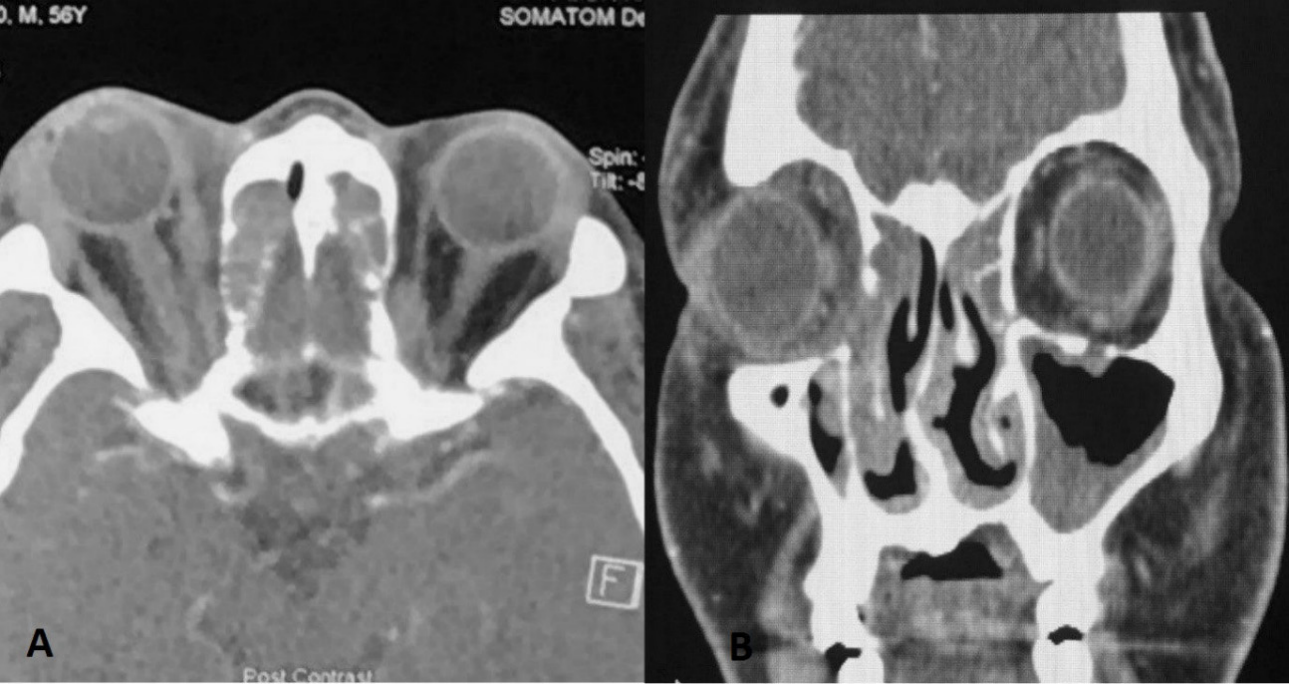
CT scan imaging of the orbit and paranasal sinuses: A) Axial view (left) demonstrated right periorbital soft tissue swelling with right extraocular muscle bulkier compared to left side. There was enlargement of the right lacrimal gland. Soft tissue density was seen within ethmoidal air cells. B) Coronal view (right) demonstrated soft tissue density in the frontal sinus, ethmoidal air cells and mucosal thickening in both the maxillary sinuses with obliteration of both osteomeatal complex. There was erosion of the right lamina papyracea.
